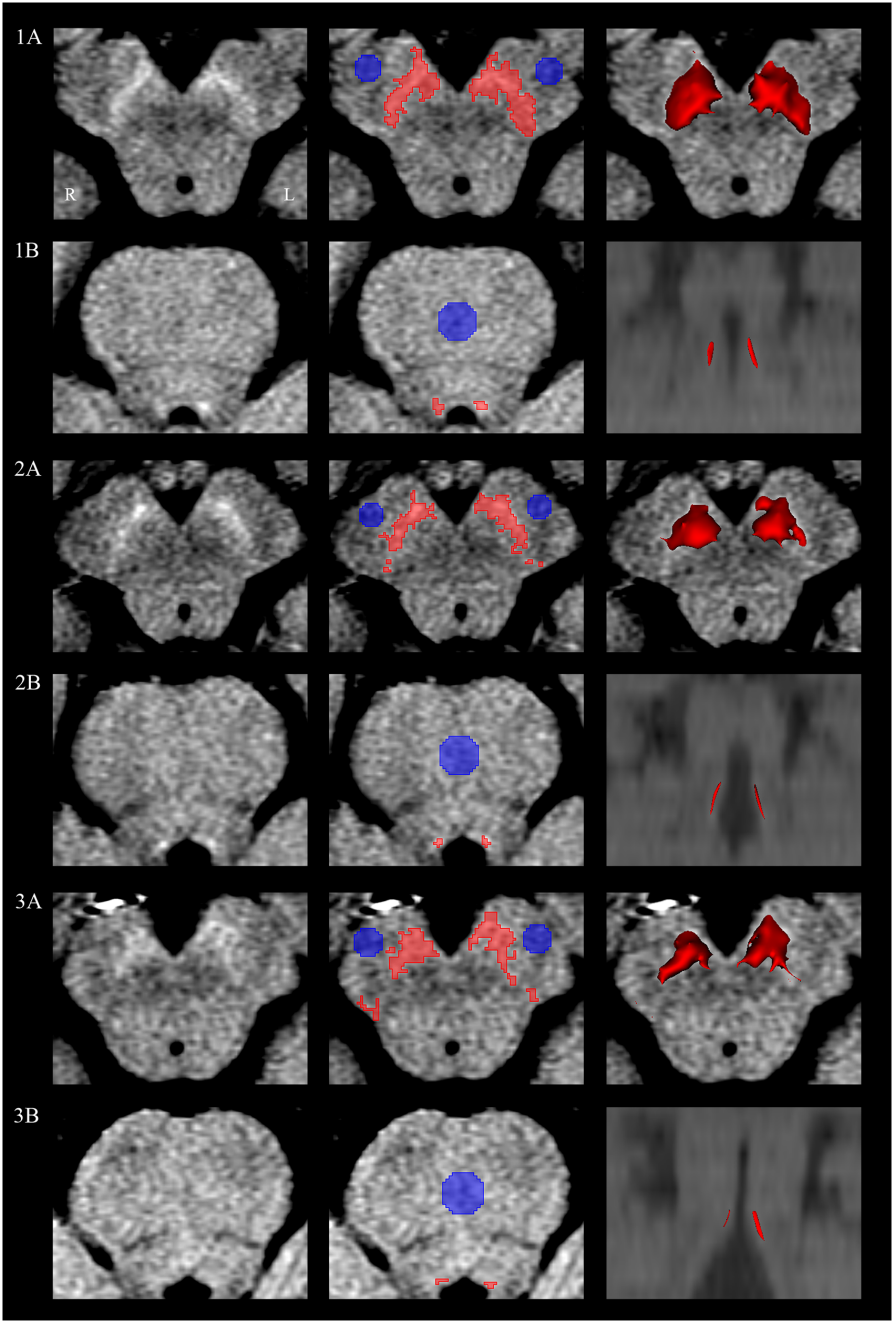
Semiautomated interactively segmented regions of interest on neuromelanin MRI. Substantia nigra and locus coeruleus of a HC subject (1A,1B), PD-NFOG patient (2A,2B) and PD-FOG patient (3A,3B). Neuromelanin MRI images of the substantia nigra and locus coeruleus (first column). Regions of interest of substantia nigra and locus coeruleus detected in the semiautomated segmentation shown in red, background reference regions of interest of cerebral peduncle and pontine shown in blue (second column). Three-dimensional surface rendering corresponding to semiautomated segmentation of the bilateral substantia nigra and locus coeruleus (third column). L, left; MRI, magnetic resonance imaging; R, right.
